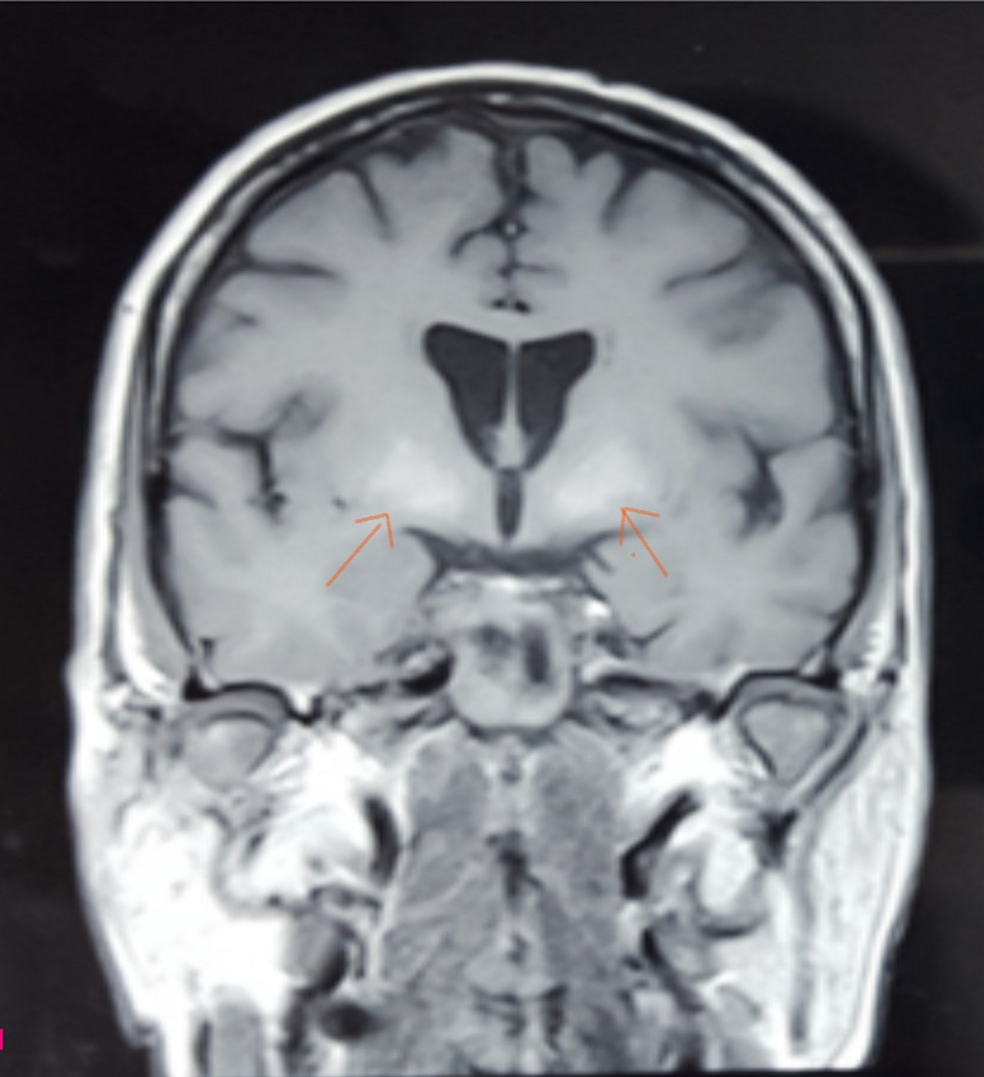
Magnetic resonance imaging coronal view of the brain showing T1 weighted image revealing hyperintensity in bilateral basal ganglia due to mineral deposition. Both the arrows point out to the hypertense foci at the basal ganglia bilaterally on a T1-weighted MRI image that suggests mineral deposition.
